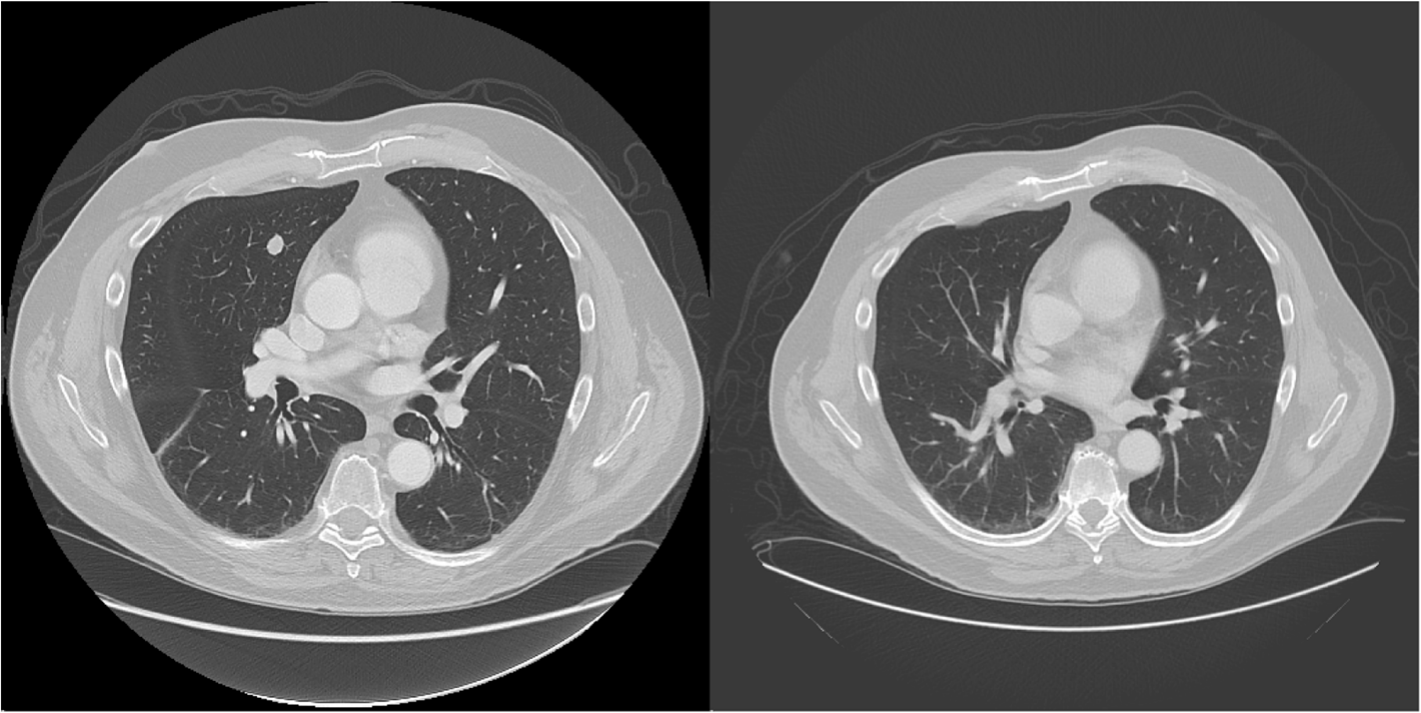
Resolution of Metastatic Pulmonary Nodule after Treatment with Pembrolizumab, (Left) CT chest from 9/22/2017 revealing RML nodule 1.2 × 1.0 cm in size. (Right) CT chest from 12/14/2017 demonstrating interval resolution of RML nodule after 4 cycles of pembrolizumab
